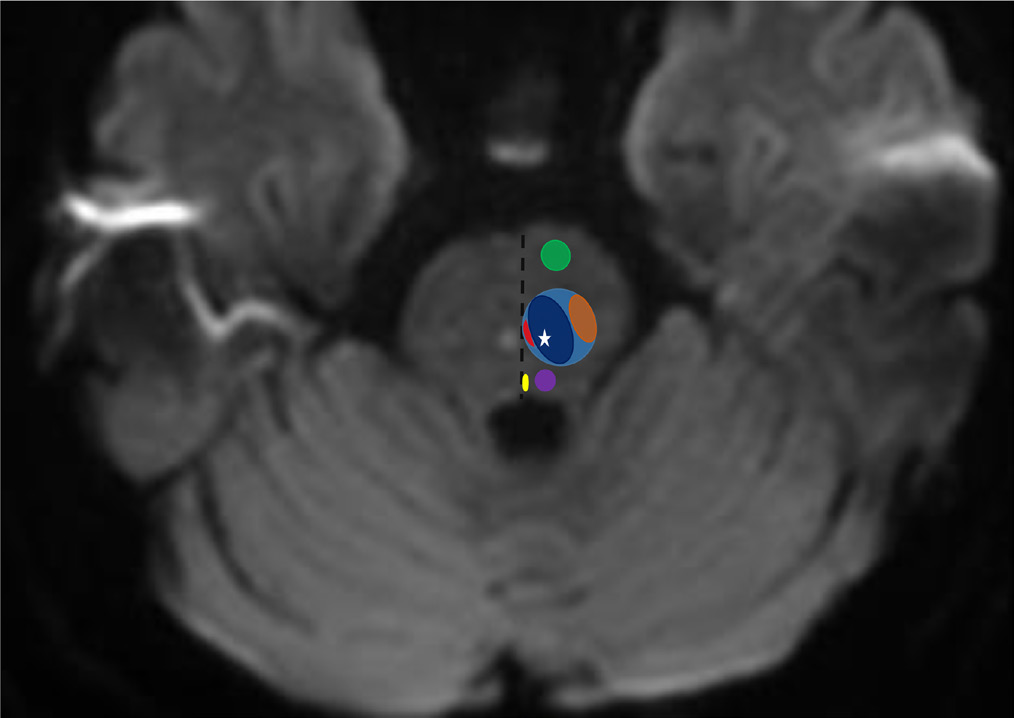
Axial MRI section at the level of pons showing two bilateral symmetric foci of diffusion restriction involving reticular formation region, with a scheme of related pontine regions in the left hemi pons. Descending pyramidal tracts (green oval), medial longitudinal fasciculus (yellow oval), central tegmental tract (purple oval), reticular formation (light blue oval) containing raphe nucleus (red oval), gigantocellular nuclei (dark blue oval) and parvocellular nuclei (orange oval). The hypreintense lesion in the left reticular formation (white star) is likely corresponding to the medial pontine nuclei (gigantocellular nuclei).
